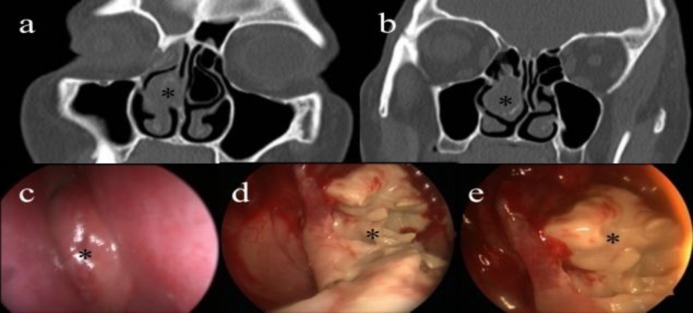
a,b) Paranasal computed tomography image of the infected concha bullosa; c) pre-operative image of the middle turbinate; d,e) View of fungal material in the intra-operative concha bullosa
